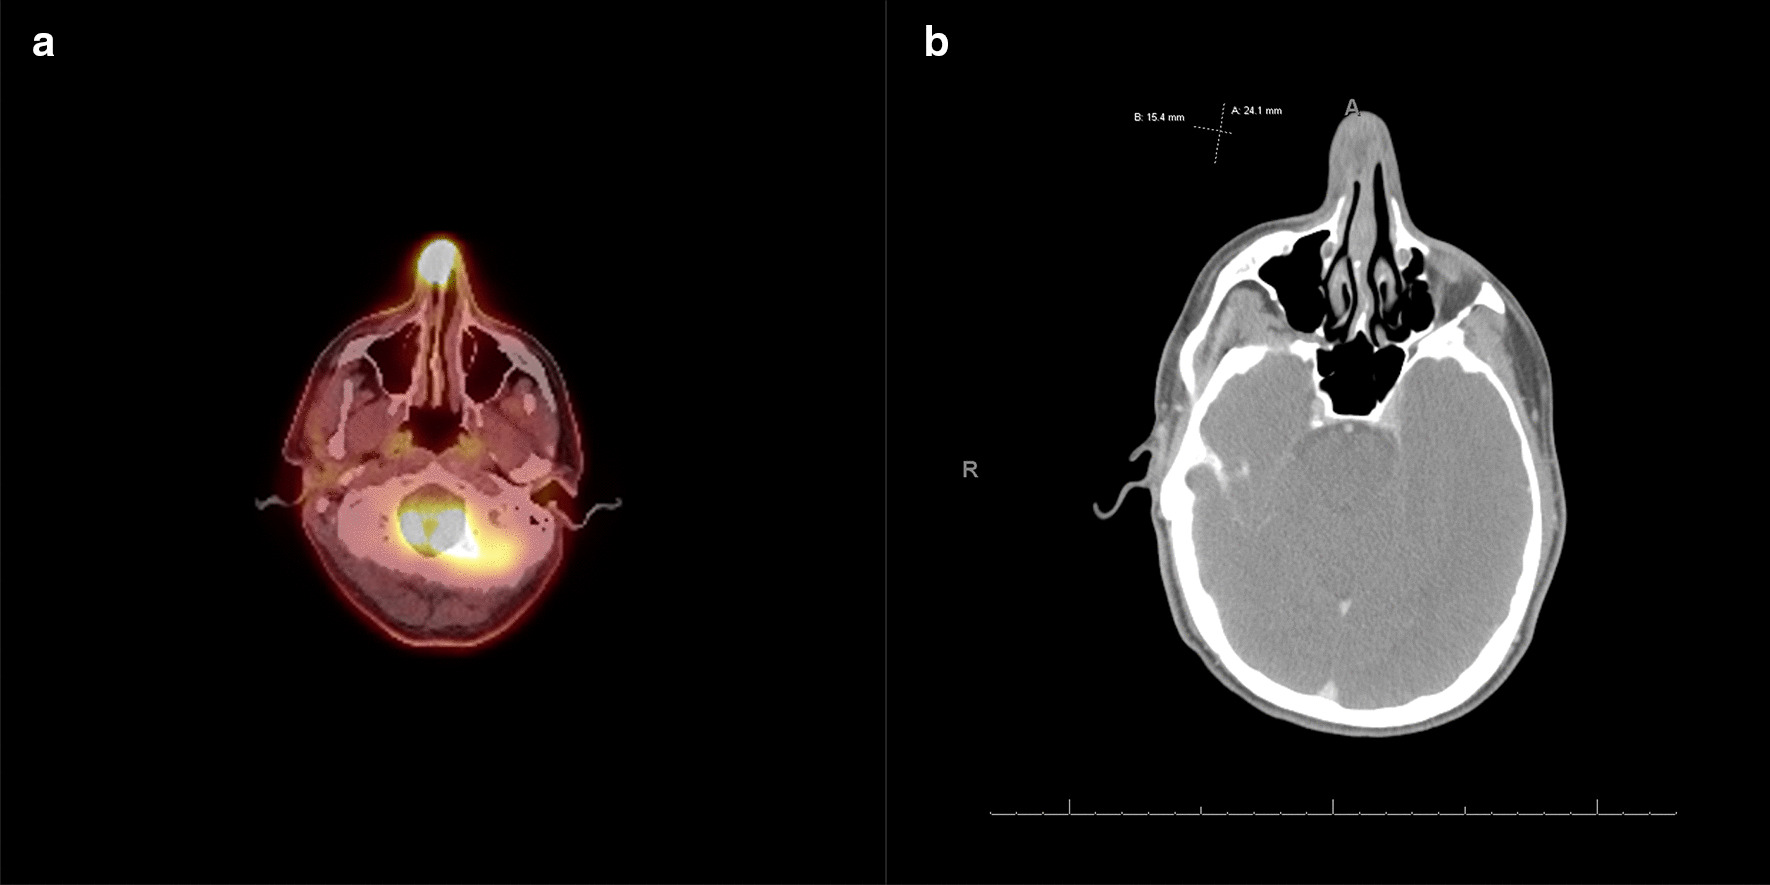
Patient imaging findings including head and neck a positron emission tomography–computed tomography (PET-CT) and b CT with contrast showing 15.4 mm × 24.1 mm right nasal mass and local disease only
